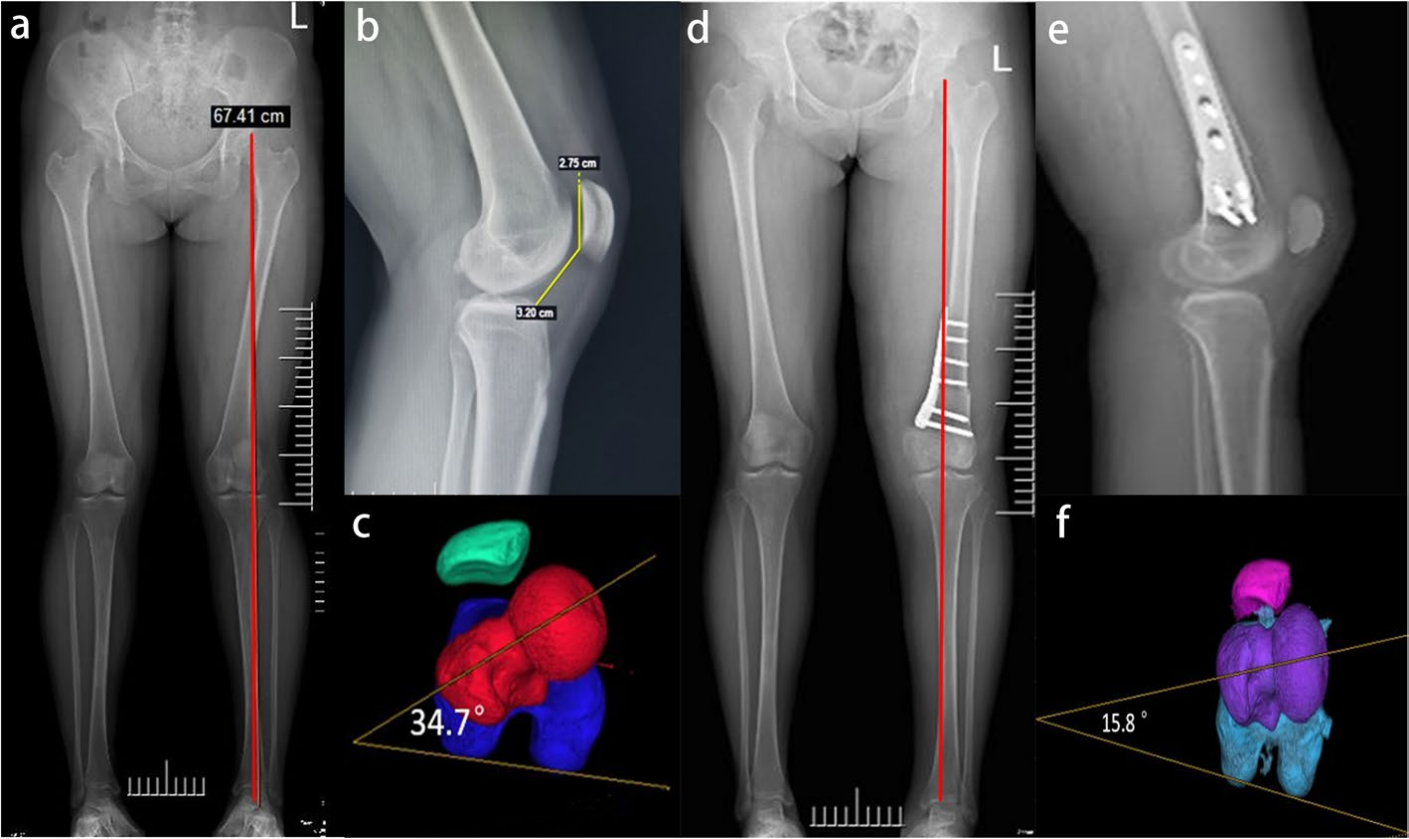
Genu valgum deformity with recurrent patellar dislocation of a 19-year-old female. a Preoperative full-leg standing anteroposterior radiographs showed knee valgus deformity. b. Preoperative lateral radiograph. c. The preoperative femoral anteversion angle was increased to 34.7°. d. Postoperative full-leg standing anteroposterior radiographs showed neutral alignment. e. Postoperative lateral radiograph showed stable fixation with a Tomofix-locking plate. f. CT scans postoperatively showed an improvement in femoral anteversion angle
